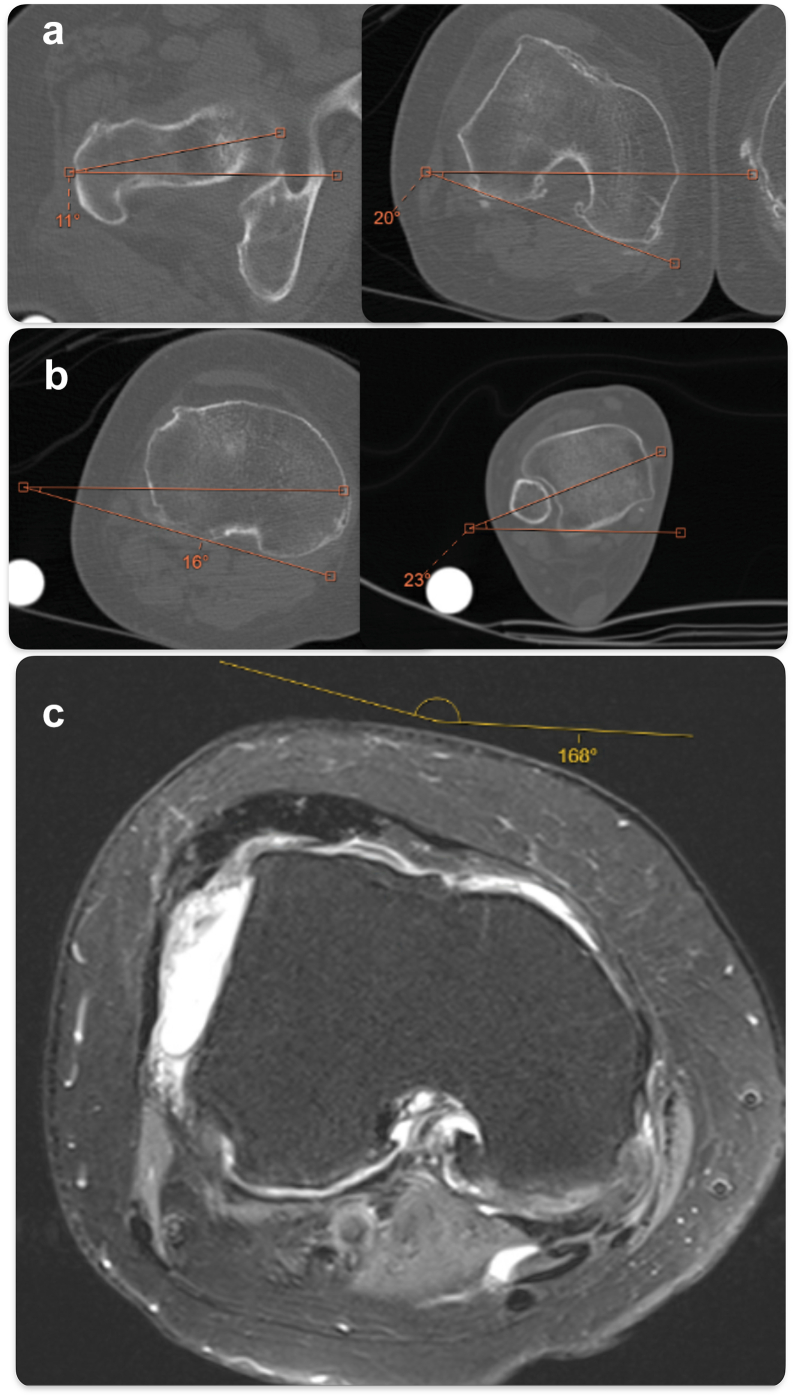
(a) CT femur anteversion, (b) CT tibial torsion, (c) MRI axial cut of the knee. Excessive femoral anteversion and tibial torsion were noted, with trochlear dysplasia on MRI and lateralization of the extensor mechanism. CT, computed tomography.
